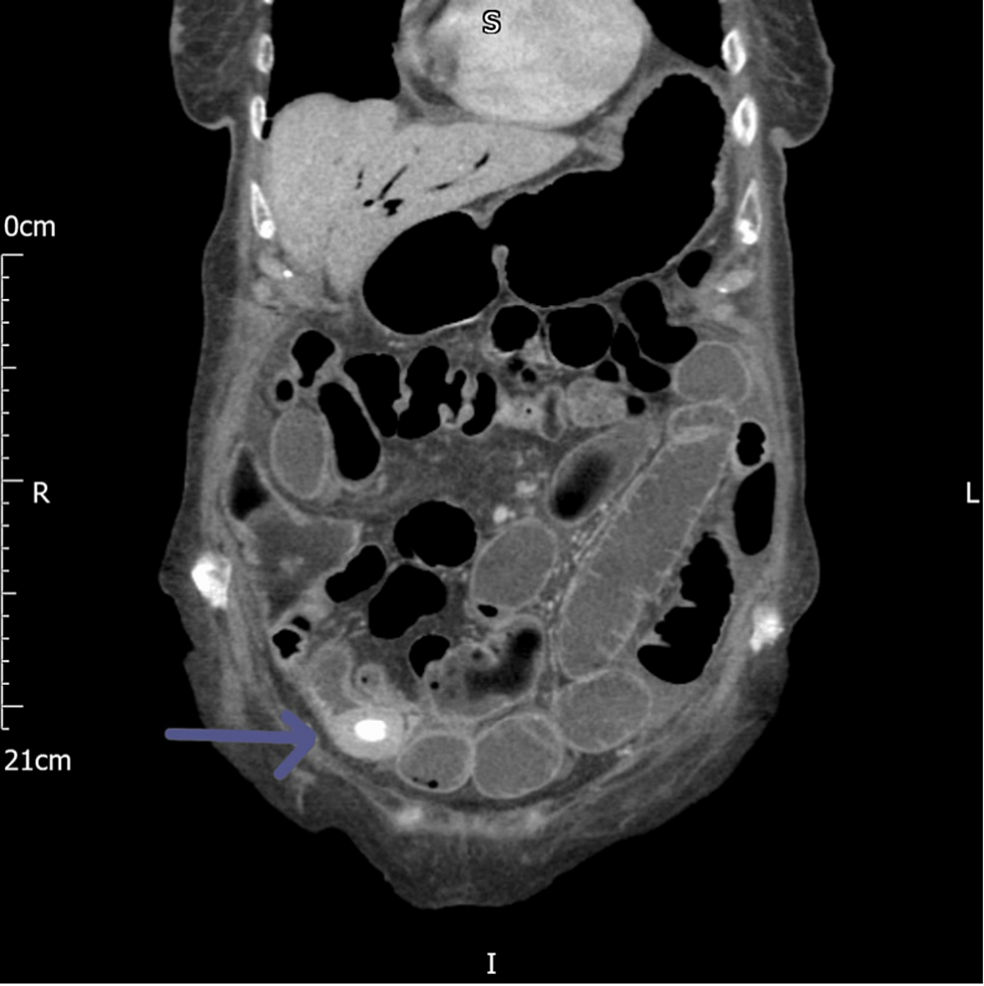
CT of the abdomen coronal view showing gallstone in the terminal ileum (the blue arrow) CT: computed tomography
